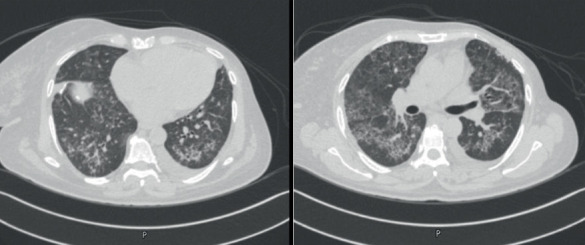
Chest CT scan demonstrated progression of GGOs compared to Figure 1.
